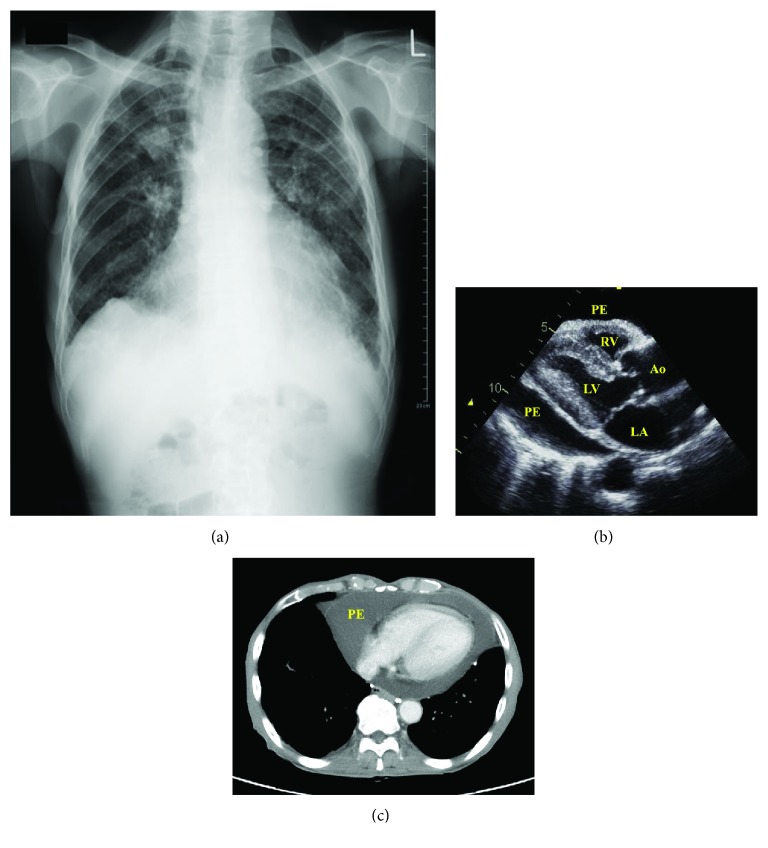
(a) Chest radiograph showing nodular opacities in the bilateral upper lung and diffuse small nodules throughout the lung field. (b) Transthoracic echocardiogram revealing a massive pericardial effusion. PE: pericardium effusion; LA: left atrium; LV: left ventricle; Ao: aorta; RV: right ventricle. (c) Computed tomography revealing a massive pericardial effusion. PE: pericardium effusion.
